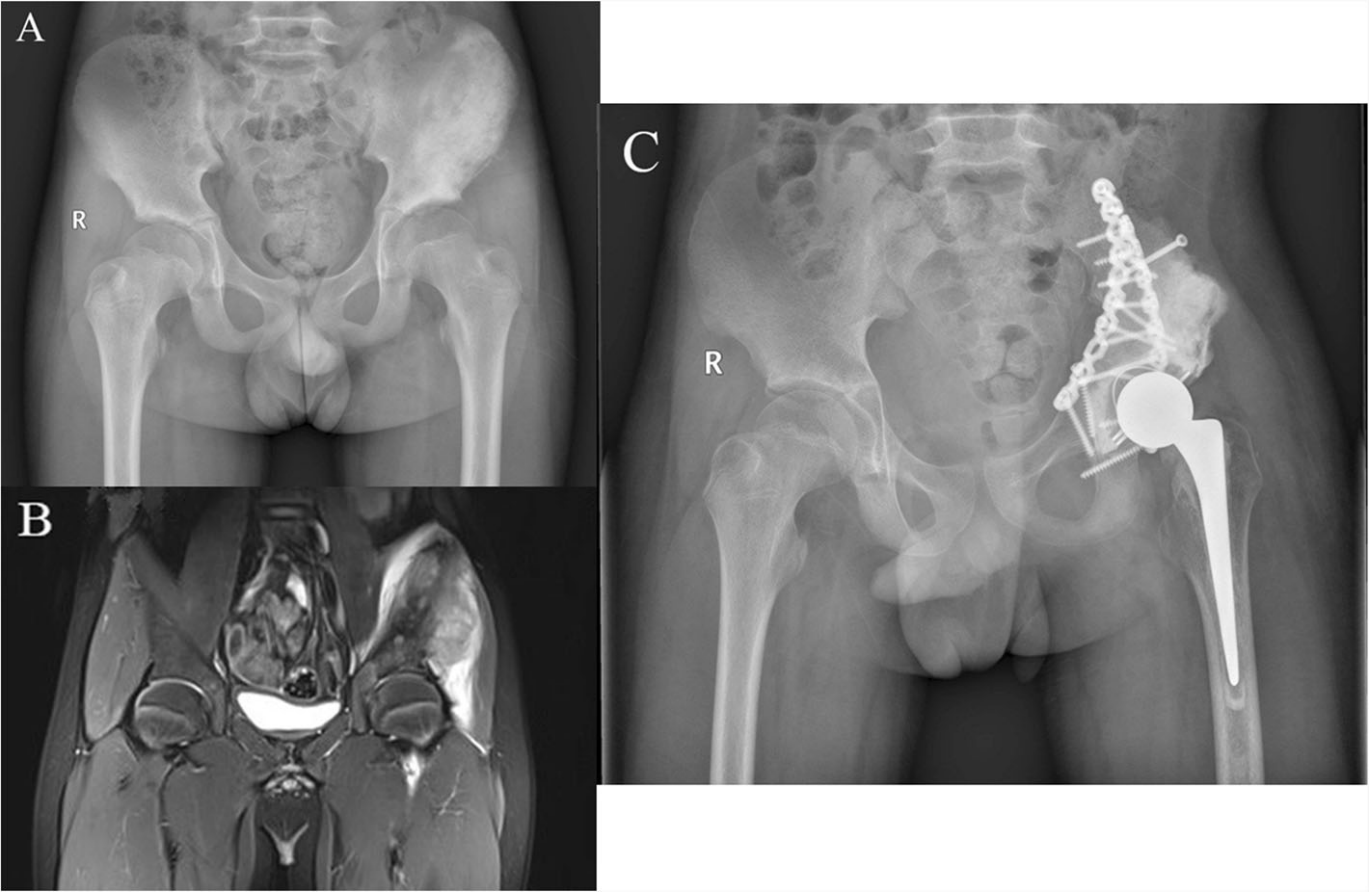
Patient 9 was a 13-year-old male with osteosarcoma. a Preoperative AP radiograph. b Preoperative MRI. c Bone union was achieved at 6 months postoperatively
